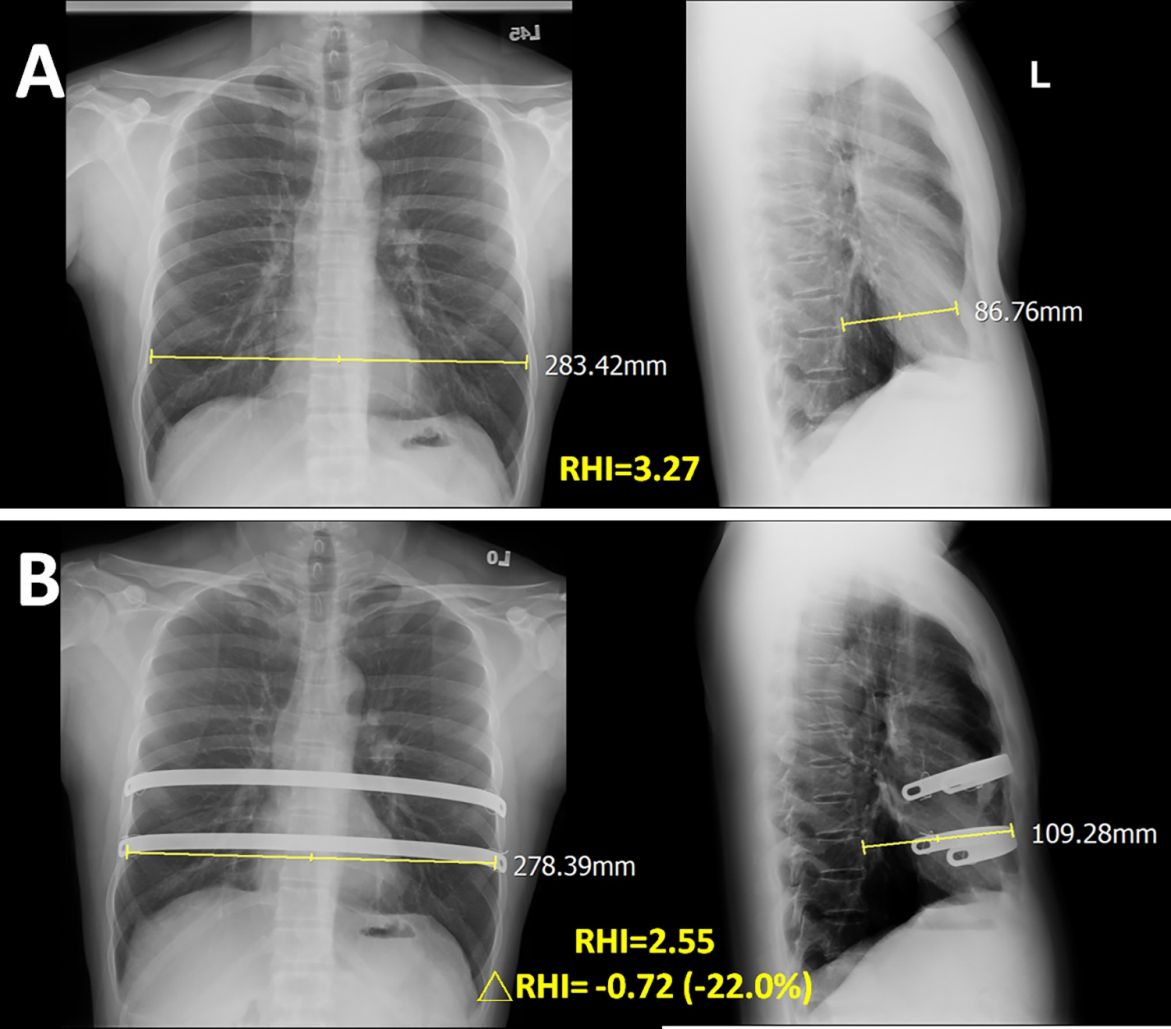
The changes of sternovertebral distance and radiographic Haller index in a patient with pectus excavatum and obstructive sleep apnea. (A) Before Nuss surgery, a patient with pectus excavatum and obstructive sleep apnea a sternovertebral distance of 86.8 mm, and a radiographic Haller index (RHI) of 3.27 mm. (B) After Nuss surgery at 24 months, the sternovertebral distance increased to 109.3 mm (25.9% increase) and the RHI decreased to 2.55 (22% decrease).
